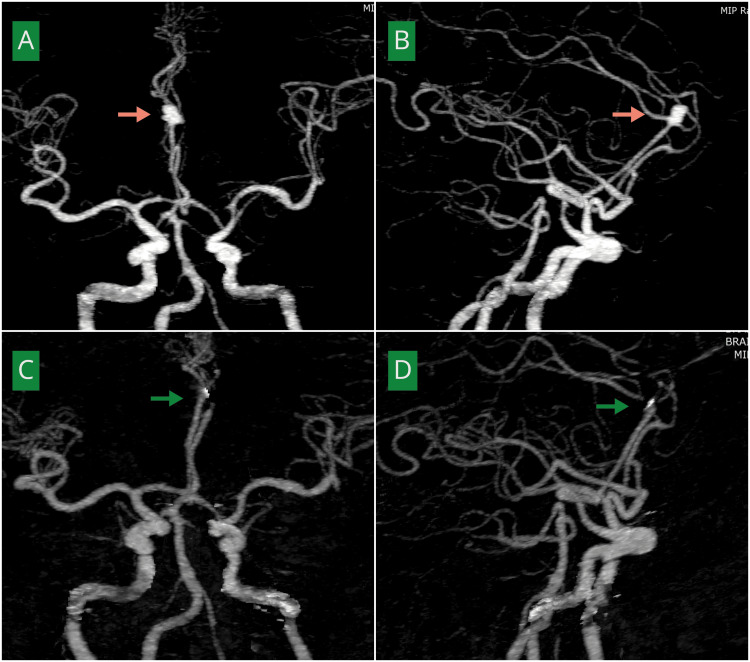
Preoperative and postoperative angiographic demonstration of microsurgical clipping of a distal anterior cerebral artery aneurysm in a 58-year-old male (A, B) Anteroposterior and lateral views of computed tomography angiography (CTA) of the brain demonstrating a right anterior cerebral artery aneurysm at the A2-A3 junction (pink arrows). (C, D) Post-clipping angiography of the same patient showing complete aneurysm occlusion with preserved distal filling of the anterior cerebral artery (green arrows).
